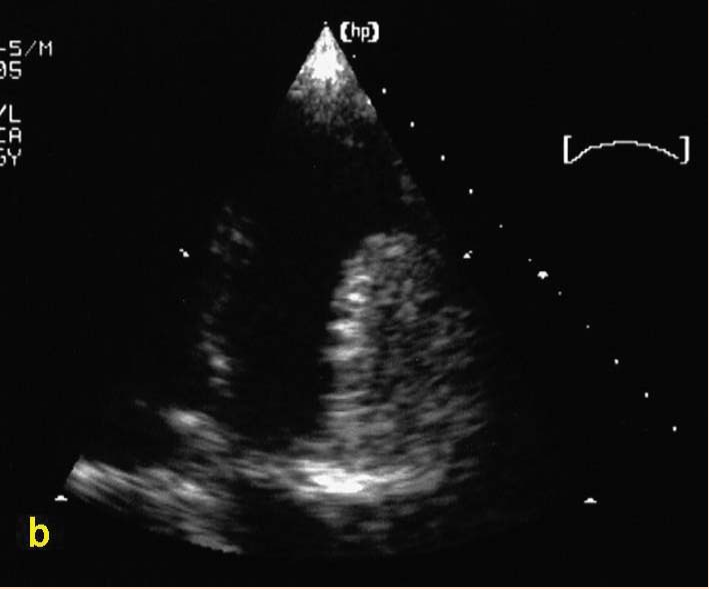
Apical four–chamber view. The ovoid tumor – homogenous and intense echostructure, near the mid / base lateral left ventricular wall and the proximal lateral left atrial wall. The tumor seems to bulge into the left ventricular cavity
